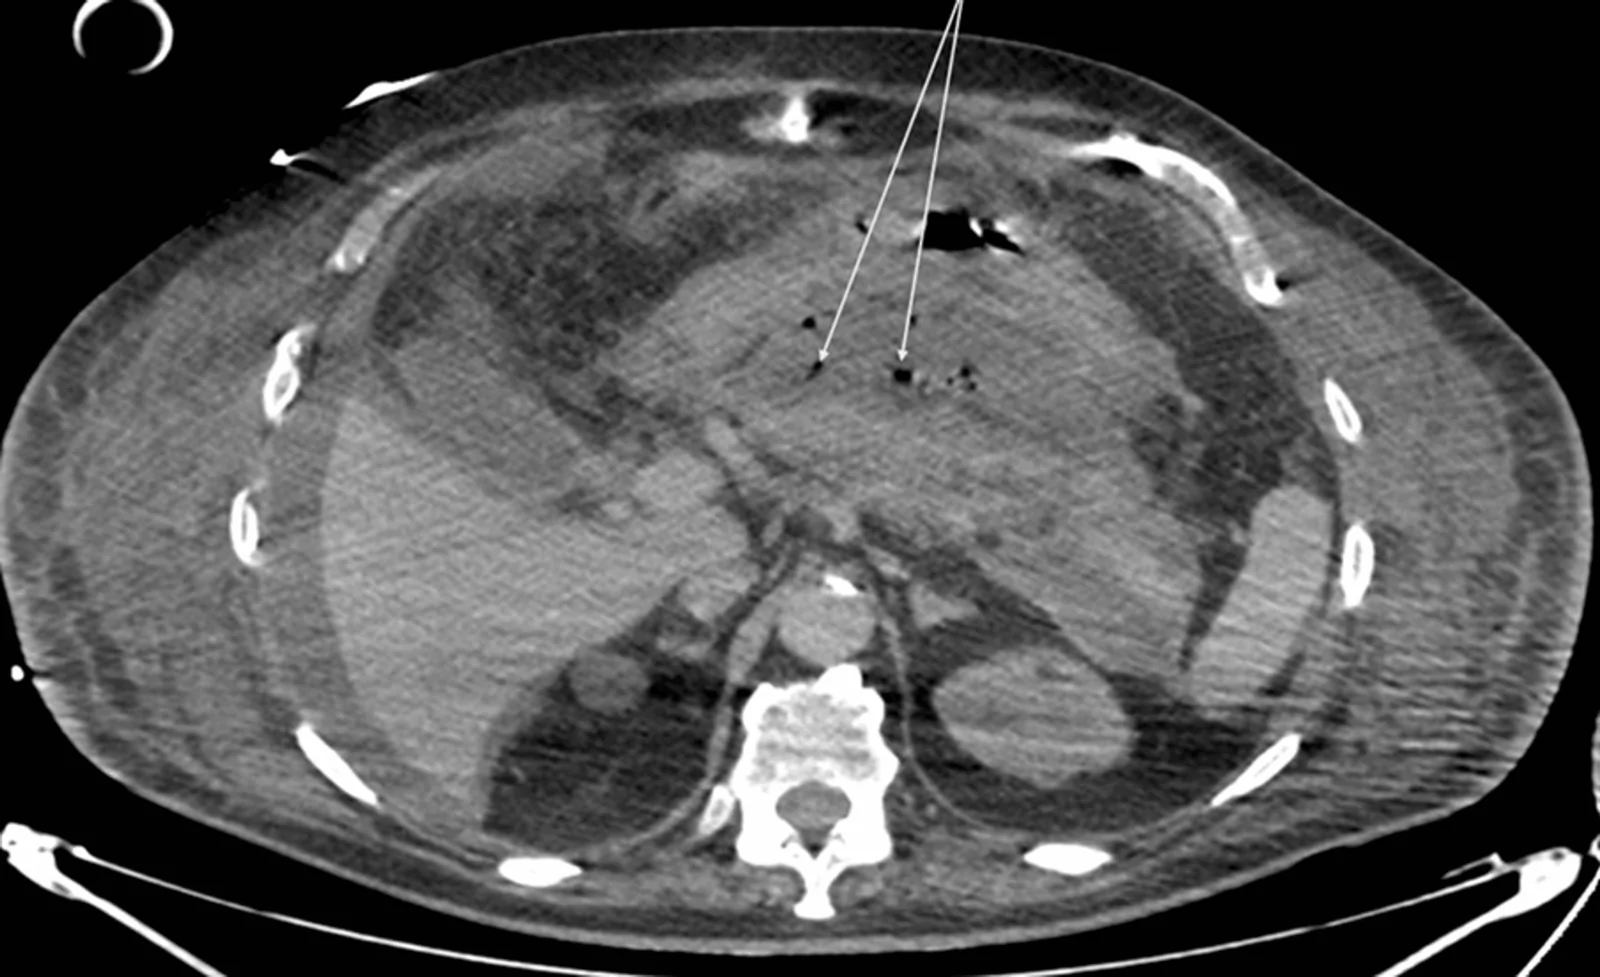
Axial contrast-enhanced abdominal CT scan upon readmission. The image demonstrates the presence of gas bubbles (arrows) within the areas of pancreatic necrosis. This finding is consistent with the diagnosis of infected pancreatic necrosis.
